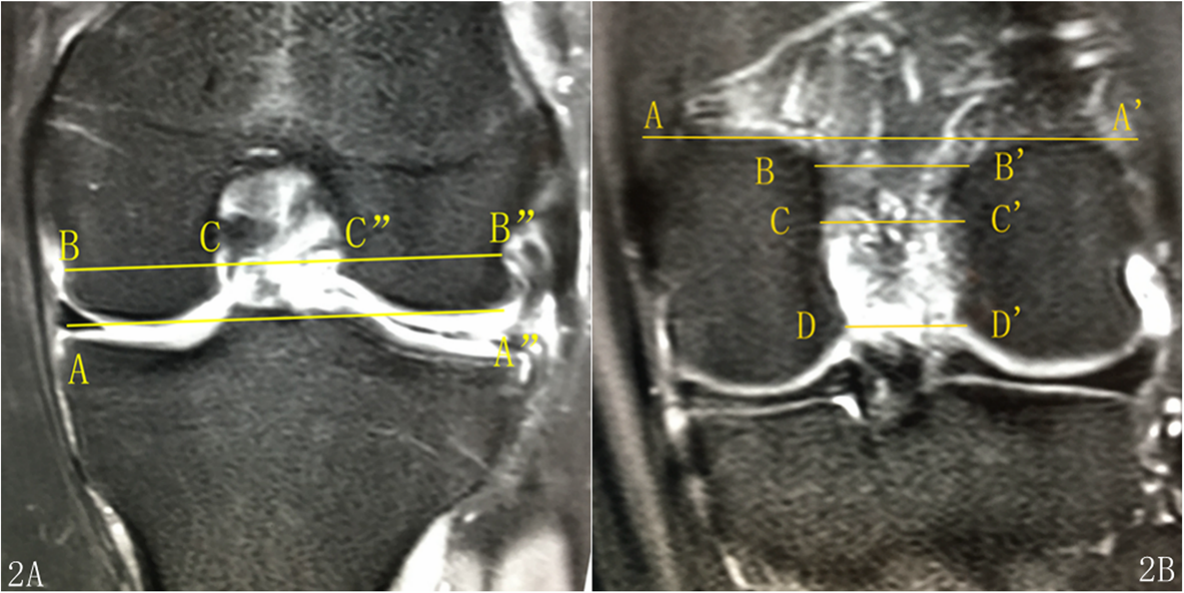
The coronal plane of the knee MRI shows the measurement of the NWs and the BW. 2A) AA” is the baseline, BB” is the BW at the level of the popliteal groove, CC” is the NW, and the NWI is equal to CC”/BB”*.* 2B) AA’ is the baseline, which is a proximal tangent of the lateral and medial femoral condyles. All the measurement of the notch width is in the same orientation as the baseline. BB’ is the NW at the notch inlet (NW_in), CC’ is the NW at the ACL attachment (NW_aa), DD’ is the NW at the notch outlet (NW_ou)
